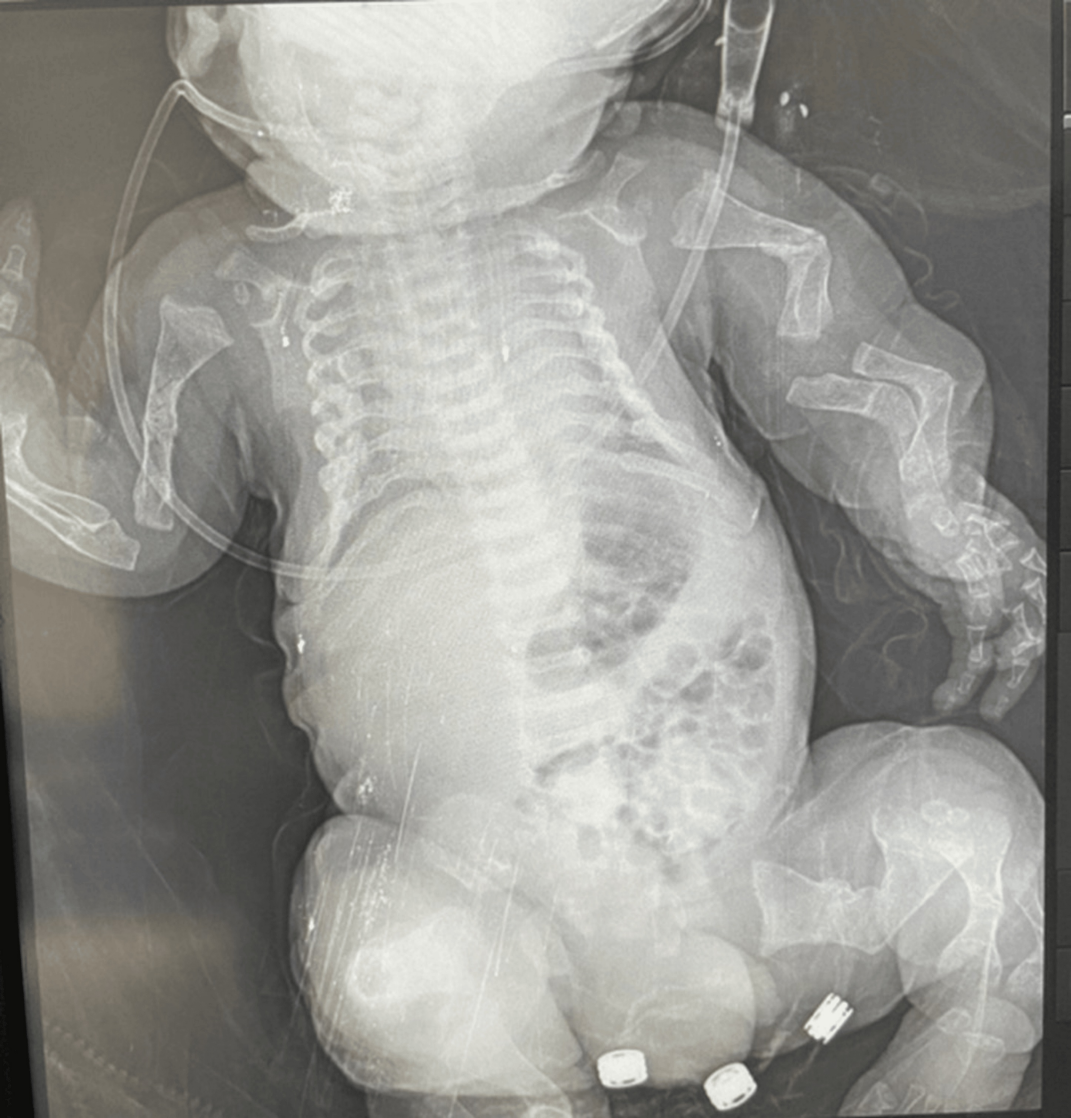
Skeletal radiograph showing markedly bowed femora with a classic “telephone-receiver” appearance, shortened ribs with a narrow thoracic cage, and generalized platyspondyly
